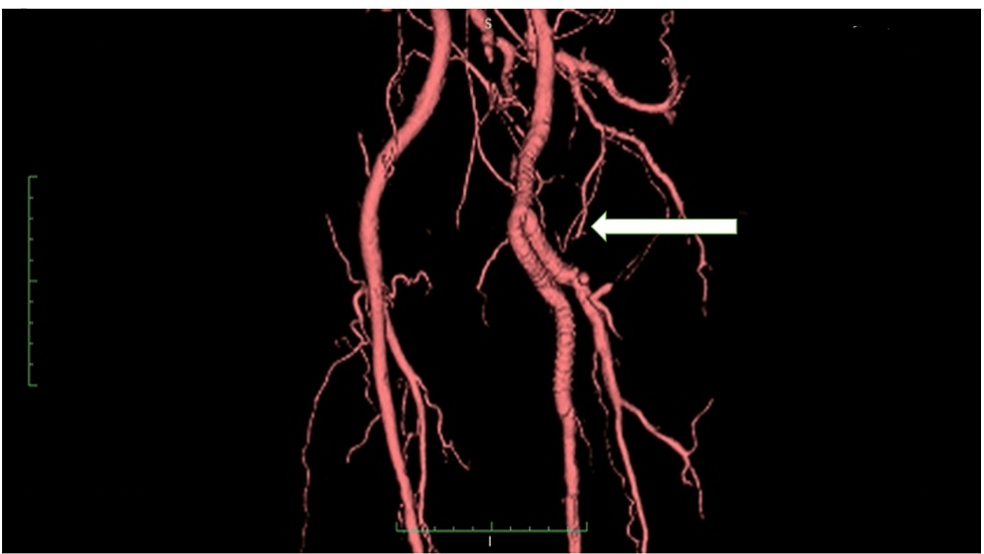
Postoperative CT No residual disease, no anastomotic stenosis (⇦). CT - computed tomography
